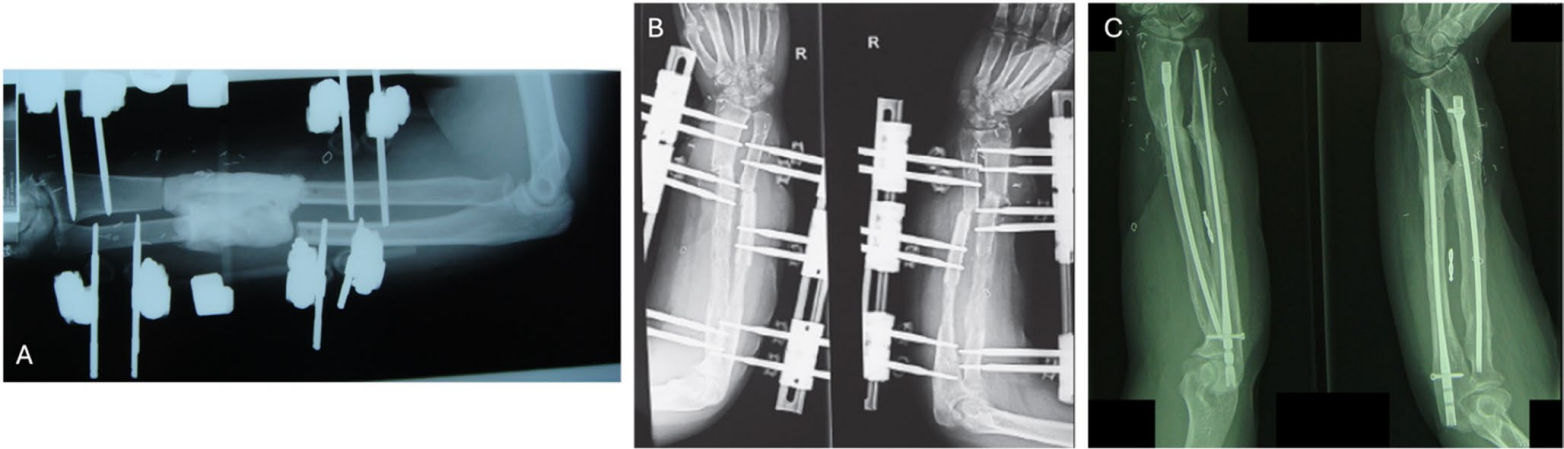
Radiographs for Case 2 A before distraction, B during distraction, and C at final outcome, with internal nails. A, B have been published previously [13]
